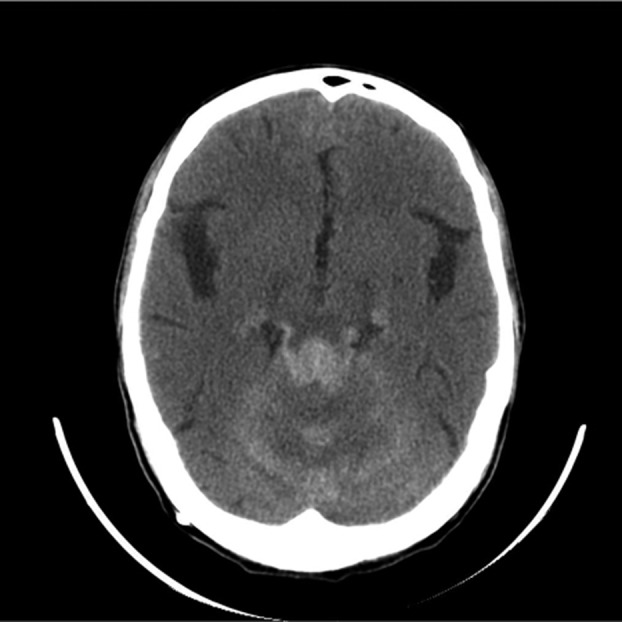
Noncontrast head CT scan is remarkable for hyperattenuated foci in basal meninges and cerebellum.
